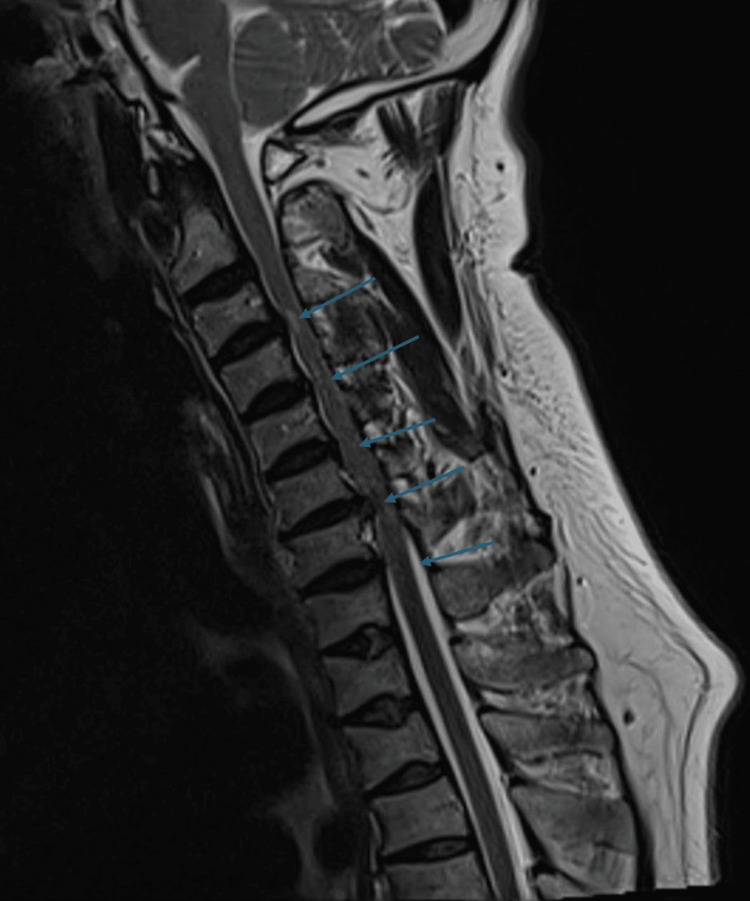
Sagittal T2-weighted MRI of the cervicothoracic spine demonstrating multilevel cervical central canal stenosis. The blue arrows indicate the sites of stenosis.
